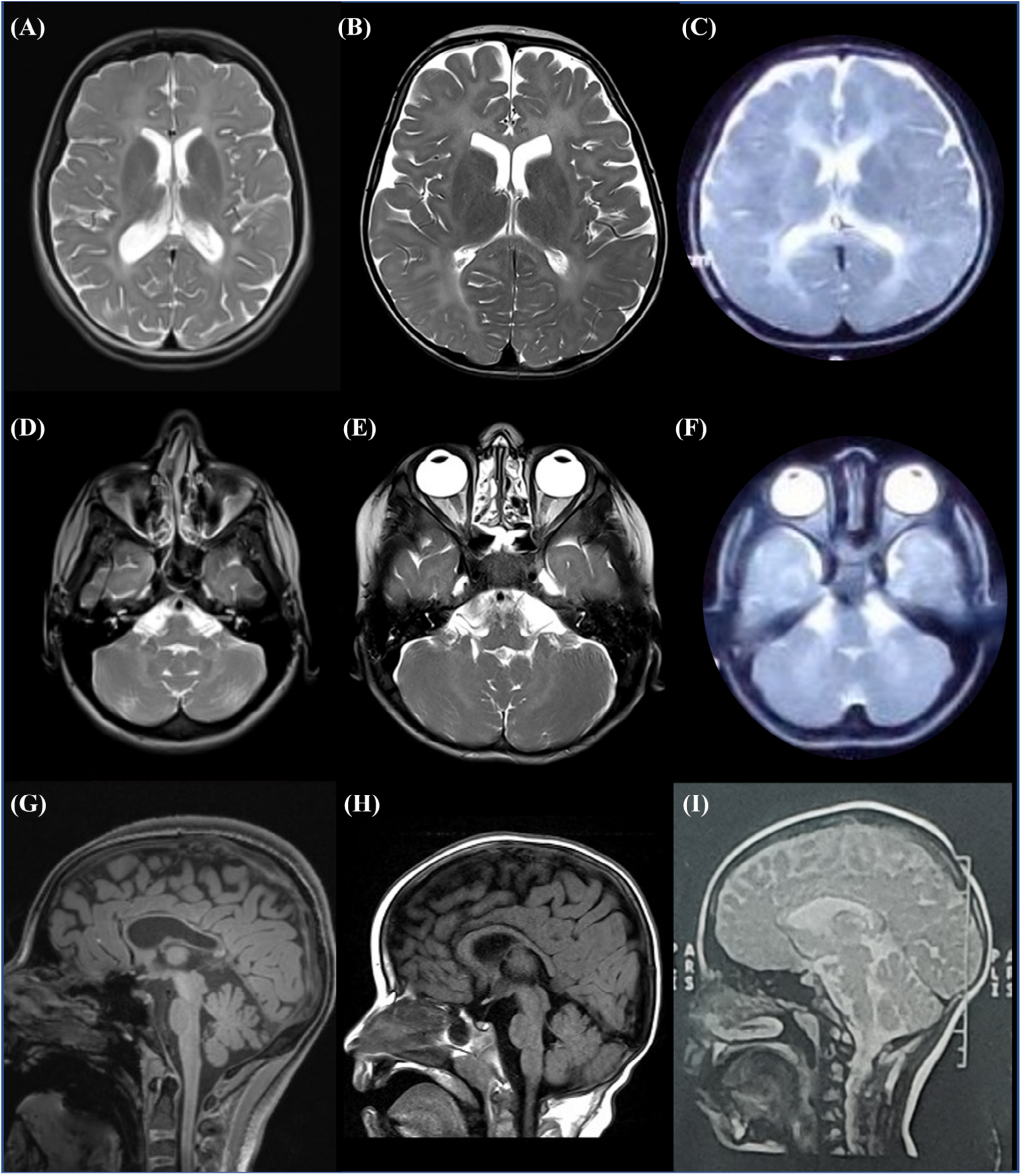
T2 weighted MR images from cases 1, 2, 4, and 5 demonstrating hypomyelination of the cerebrum (A–C) and cerebellum (C–F) and T1 weighted images showing hypoplasia of the corpus callosum (G–I). (A,D,G) Case 1 at age 14; (B,E,H) case 4 at age 5 years; (C,F) case 2 at age 6 years; and (I) case 5 at age 13 years.
